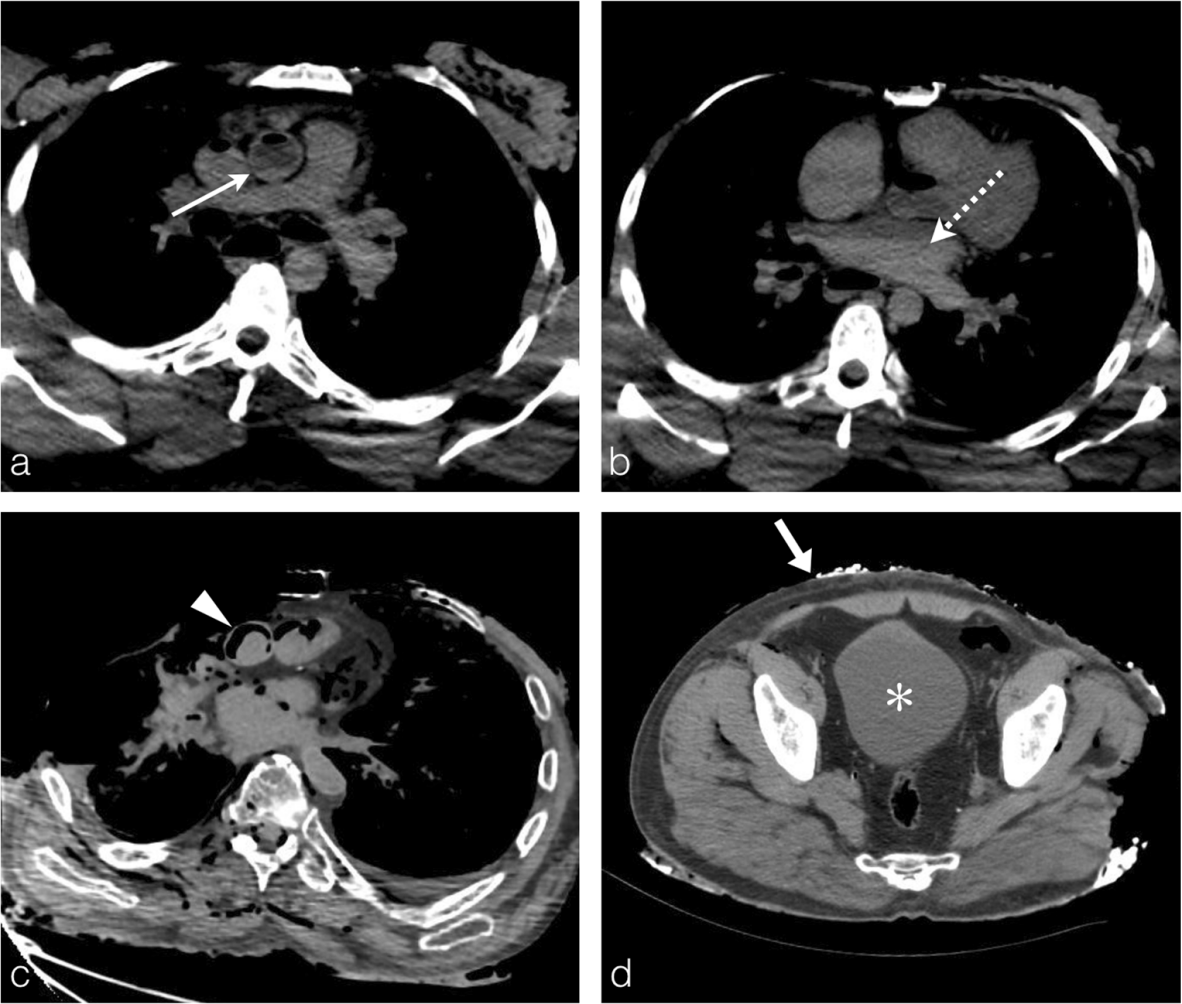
Fluid sampling. a, b A 39-year-old man who died in a fire at his home. Axial thoracic CT images viewed in the mediastinal window clearly show a fluid-fluid level in the ascending aorta (*white arrow*) and left atrium (*white dashed arrow*), pointing out non-coagulated blood available for sampling and toxicological analysis. c The severely burned body of an 89-year-old man. Axial thoracic CT image showing a thermal pericardial disrupture with exposure of the ascending aorta and cardiac cavities. An hyperdense round image is clearly identified in the lumen of the ascending aorta surrounded by a fine aeric crescent corresponding to a heat-related clot (*arrowhead*). There are also no fluid-fluid levels in the cardiac cavities or mediastinal vessels, suggesting the presence of thermally induced blood coagulation, and hence eliminating the possibility for blood sampling. d Same body as c: axial plane pelvic CT image showing a full bladder suitable for urine sampling (*asterisk*) through moderate loss of abdominal skin protecting the bladder. Note the asymmetry of fire-related injuries, with the thorax displaying multiple significant chest wall injuries, and the pelvic wall exhibiting thinning and a localised area of hyperattenuation, denoting hyperdensities of burned ashes (*thick white arrow*)
